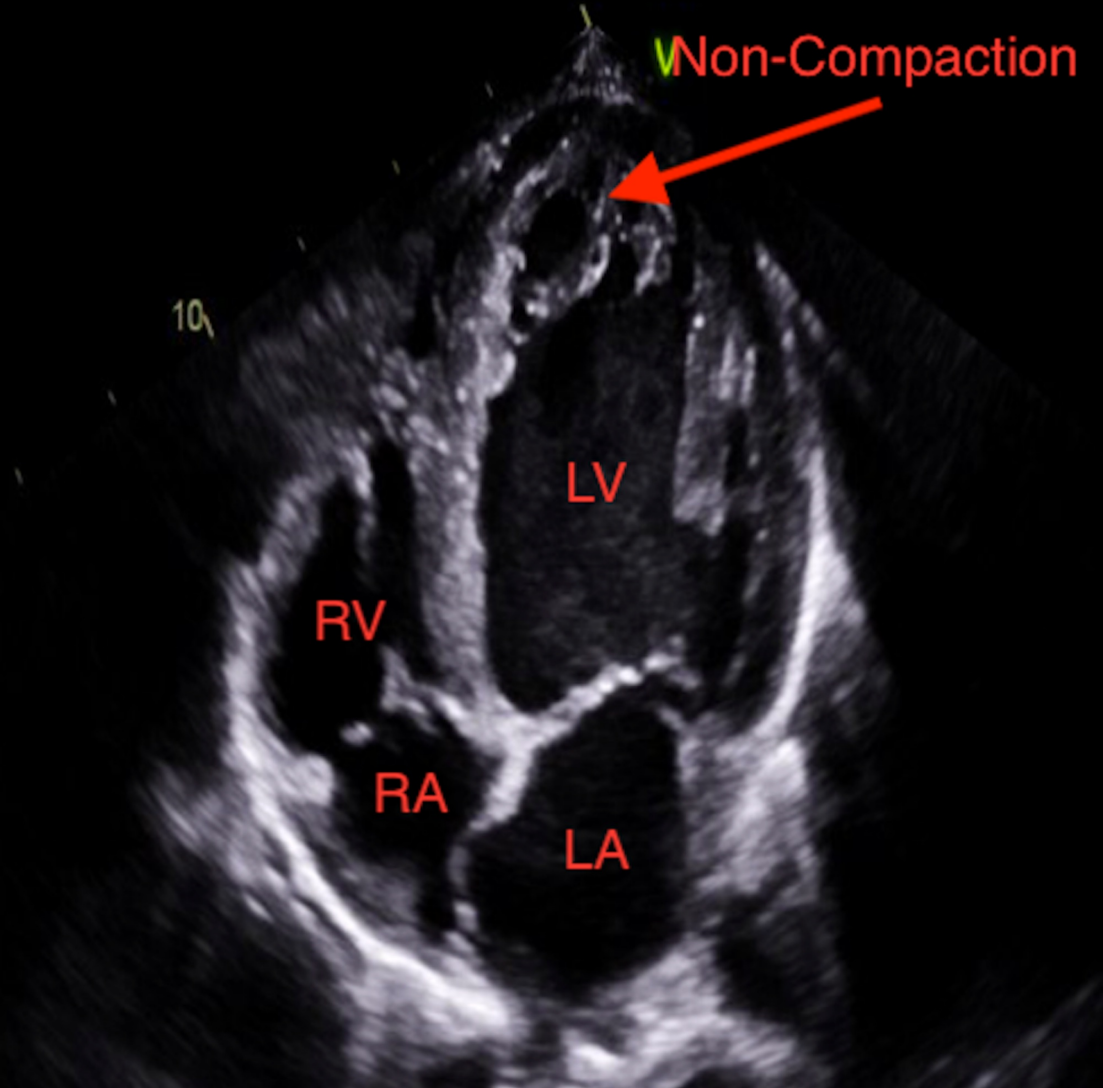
Apical 4 chamber view showing LVNC (left ventricular non-compaction). LV: Left ventricle; LA: Left atrium; RV: Right ventricle; RA: Right atrium.
